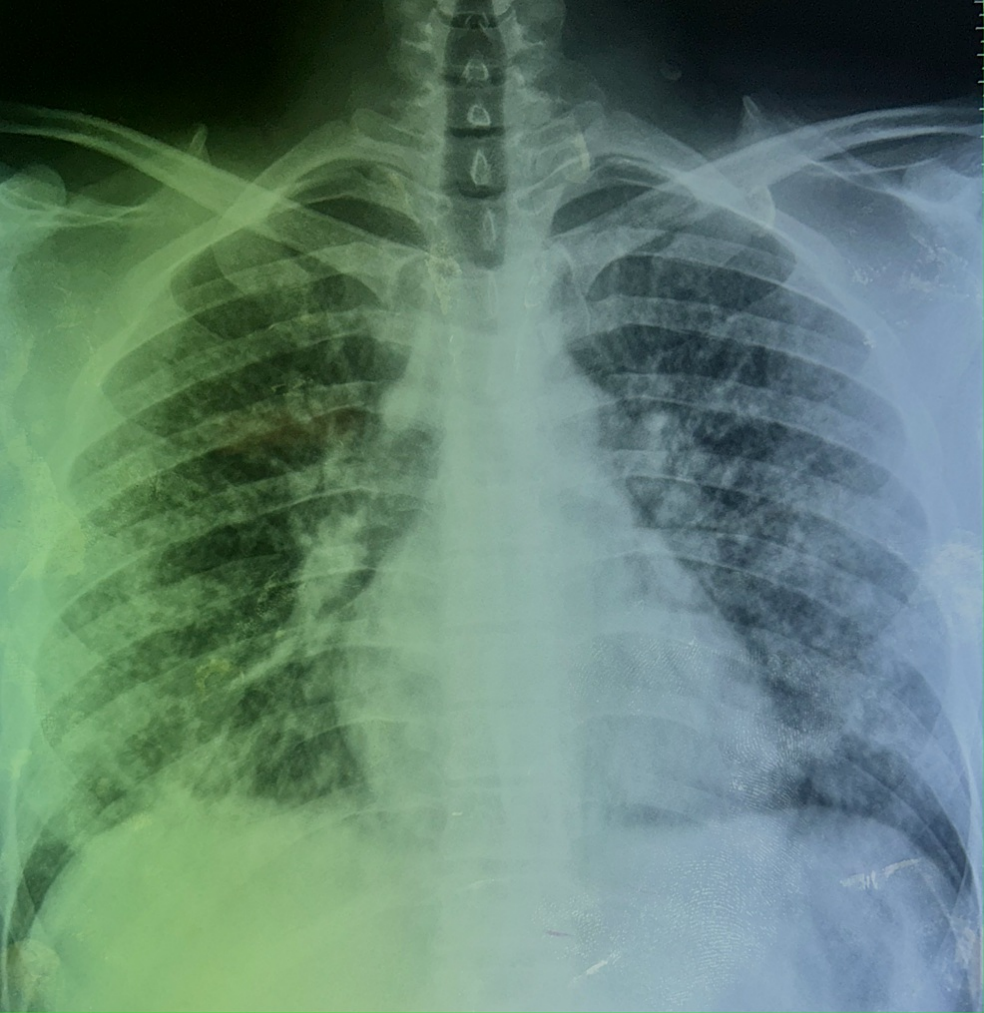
Posteroanterior chest radiograph demonstrates signs suggestive of miliary tuberculosis The image shows almost innumerable tiny interstitial nodules (e.g., 2-3 mm) distributed in both lungs some of which are confluent in places.
